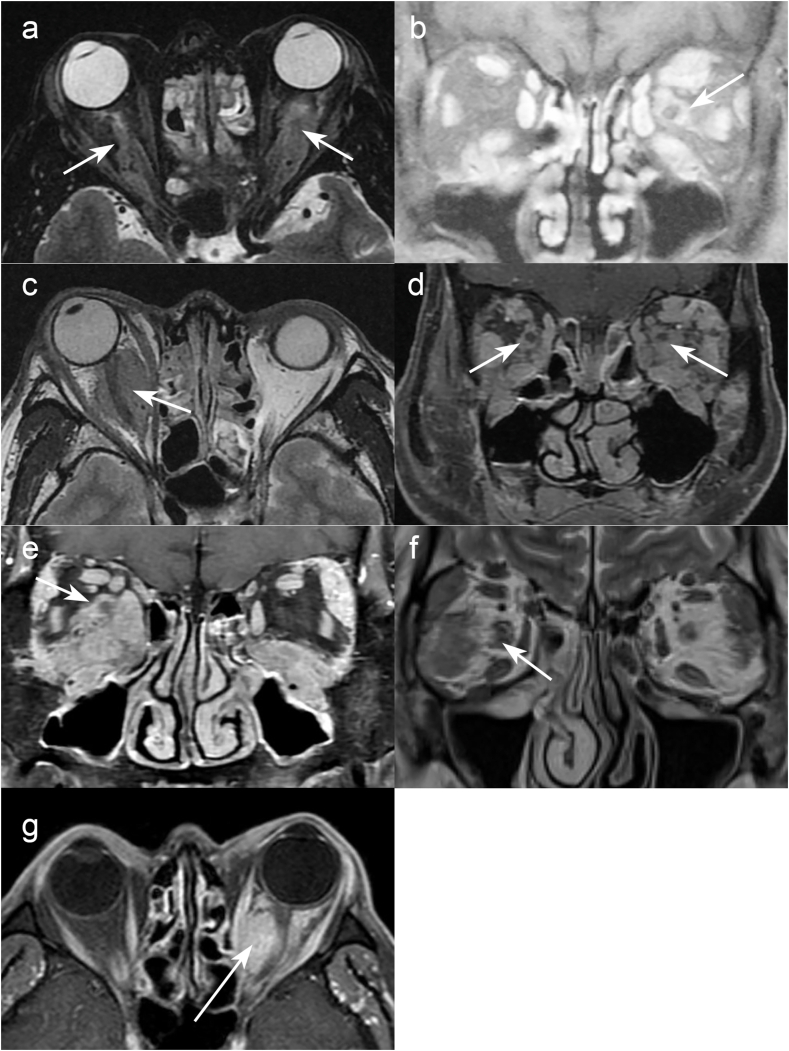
Magnetic resonance imaging (MRI) in cases with lesions surrounding the optic nerve and an orbital lesion pathology. Mass lesions around the optic nerves (arrows) were seen in seven cases of IgG4-related ophthalmic disease. All of these patients were male and the lesions were seen bilaterally around the optic nerve in case #1 (a: 52-year-old [y/o]) and case #4 (d: 57 y/o), around the left optic nerve in case #2 (b: 60 y/o) and case #7 (g: 69 y/o), and around the right optic nerve in case #3 (44 y/o), case #5 (e: 71 y/o) and case #6 (f: 44 y/o). Visual acuity was decreased due to optic neuropathy in four cases (#1, 2, 4, and 7).
